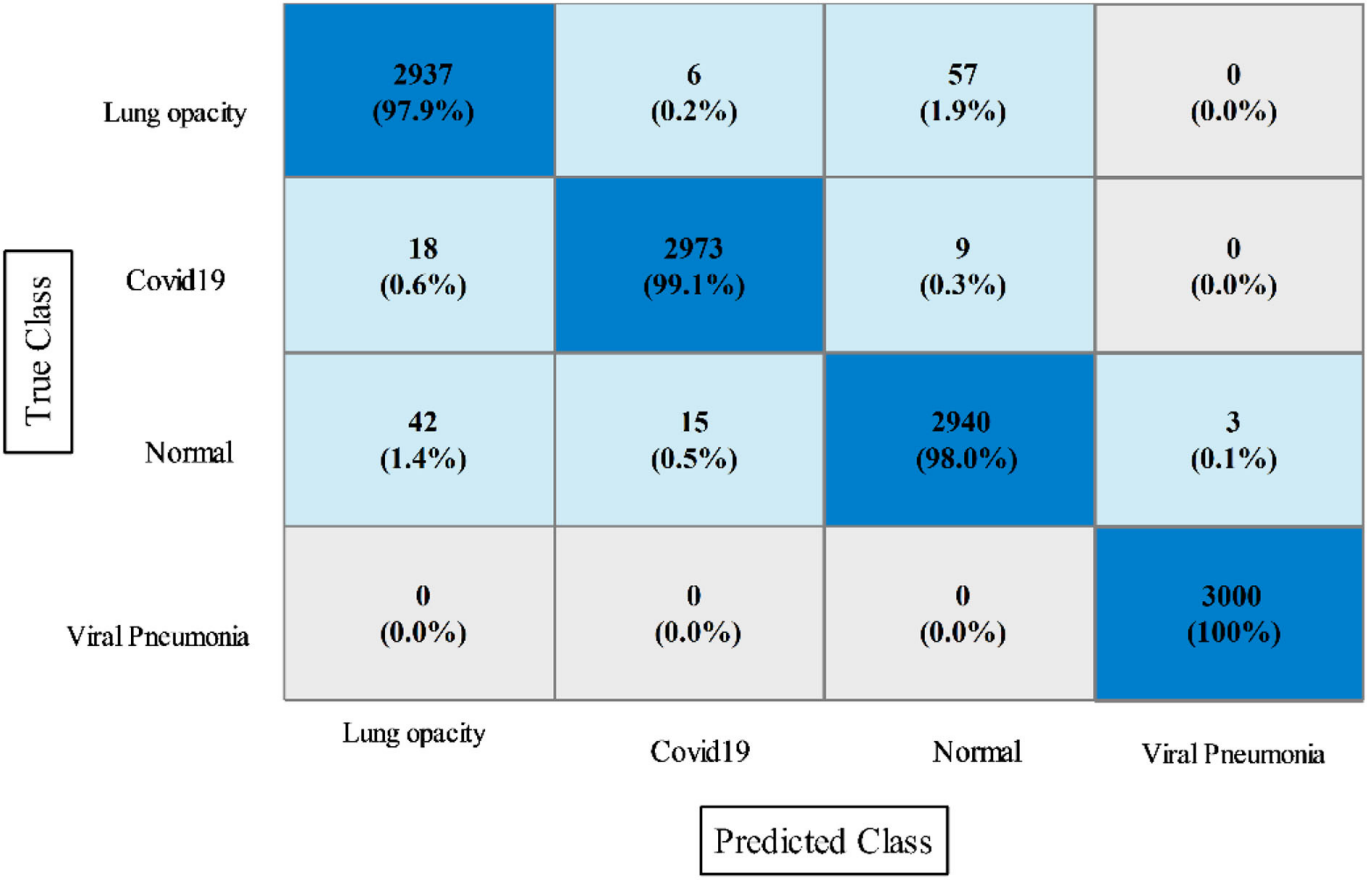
Confusion matrix of quadratic SVM utilizing the proposed slicing-based serial fusion technique on the COVID-19 radiography dataset.
